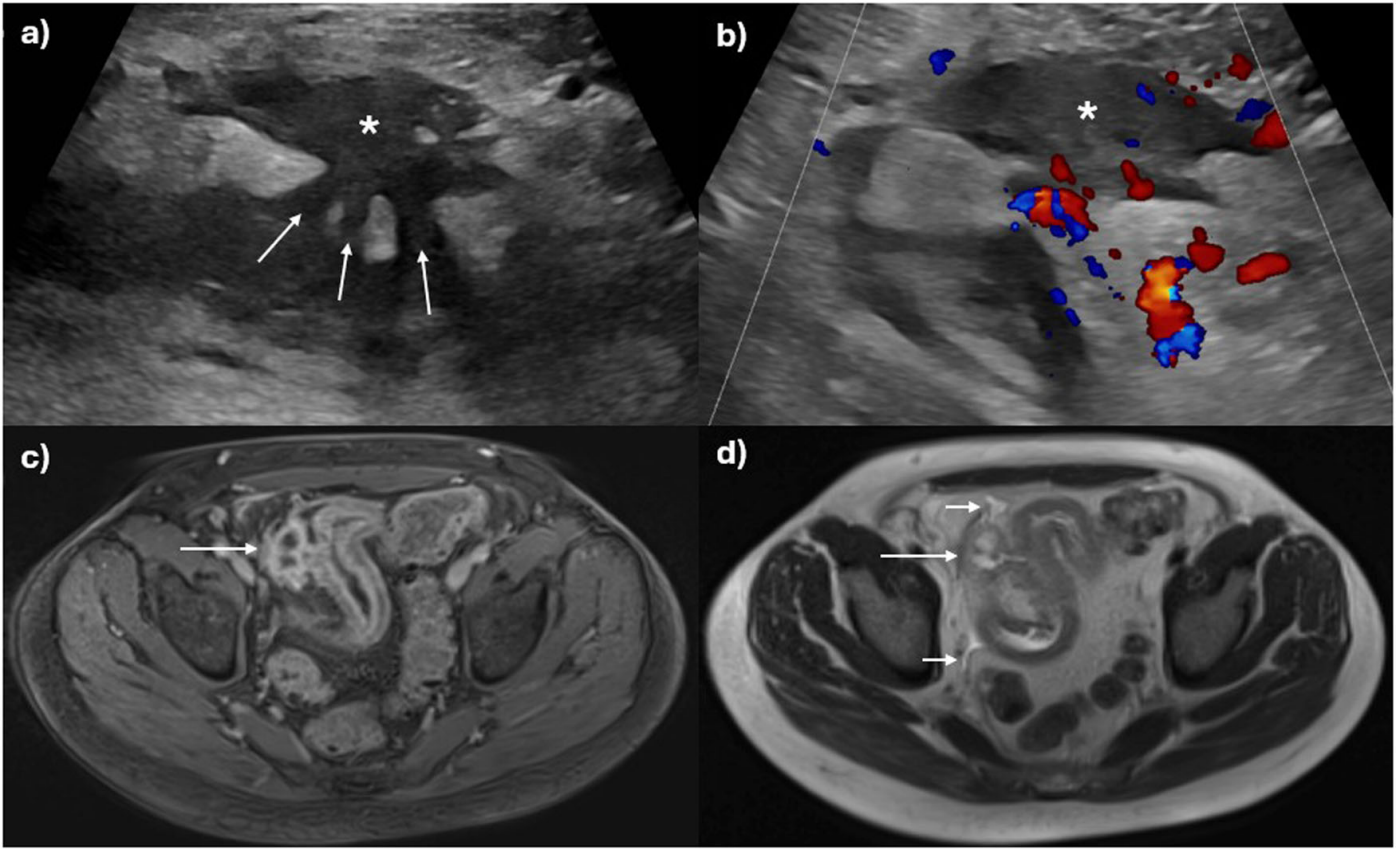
A 32-year-old male patient presents with right lower quadrant pain and elevated inflammatory blood markers following ileocaecal resection. a Ultrasound reveals several fistulous tracts (arrows) originating from the inflamed neoterminal ileum, converging into a hypoechoic inflammatory mass (asterisk) with internal vascularisation on colour Doppler imaging (b). A 41-year-old male patient with worsening symptoms and high inflammatory blood markers. c MR Enterography shows active disease in the distal ileum with layered, mural hyperenhancement on the axial fat-saturated T1-weighted post-contrast image, along with a rim-enhancing abscess (long arrow). d Axial T2-weighted image depicts small pockets of free fluid (short arrows) near the inflamed distal ileum and adjacent abscess (long arrow)
